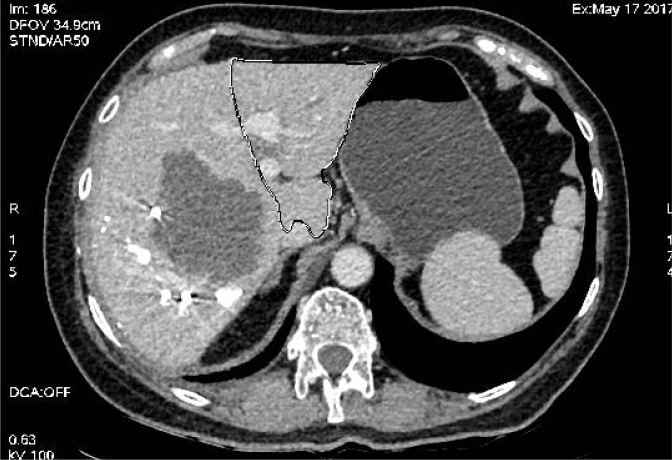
Computed tomography scan post portal vein embolization, with remnant liver volume of 22%.
